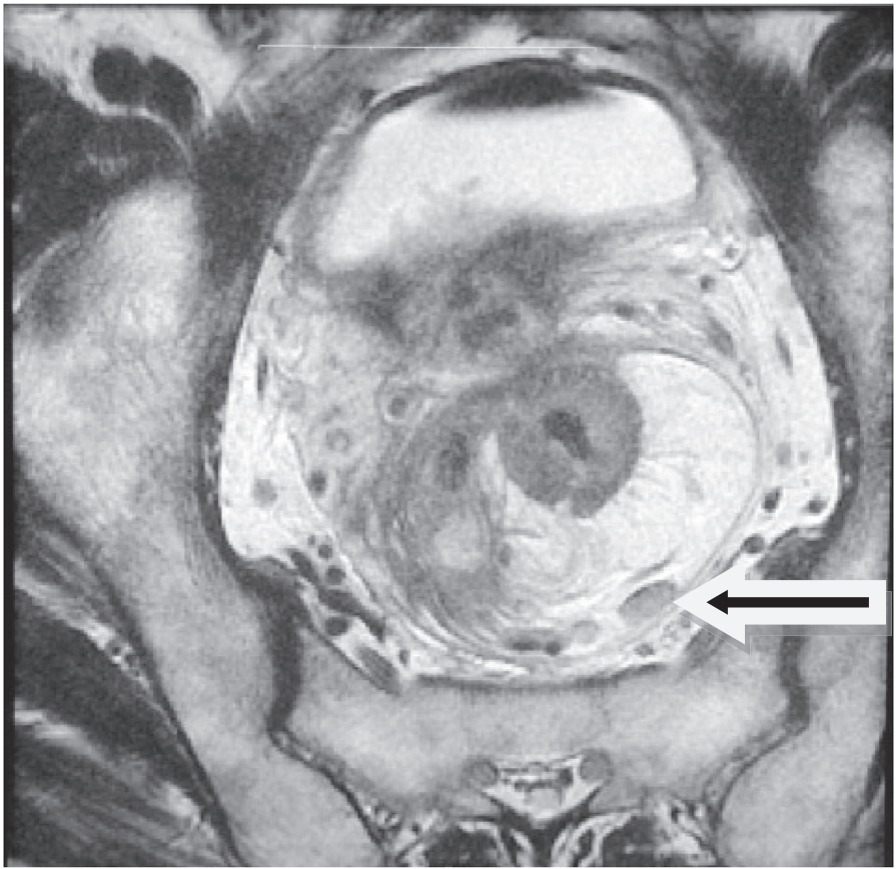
Magnetic resonance image of rectal cancer prior to neoadjuvant therapy. Arrow indicates the enlarged lymph nodes in mesorectum.
